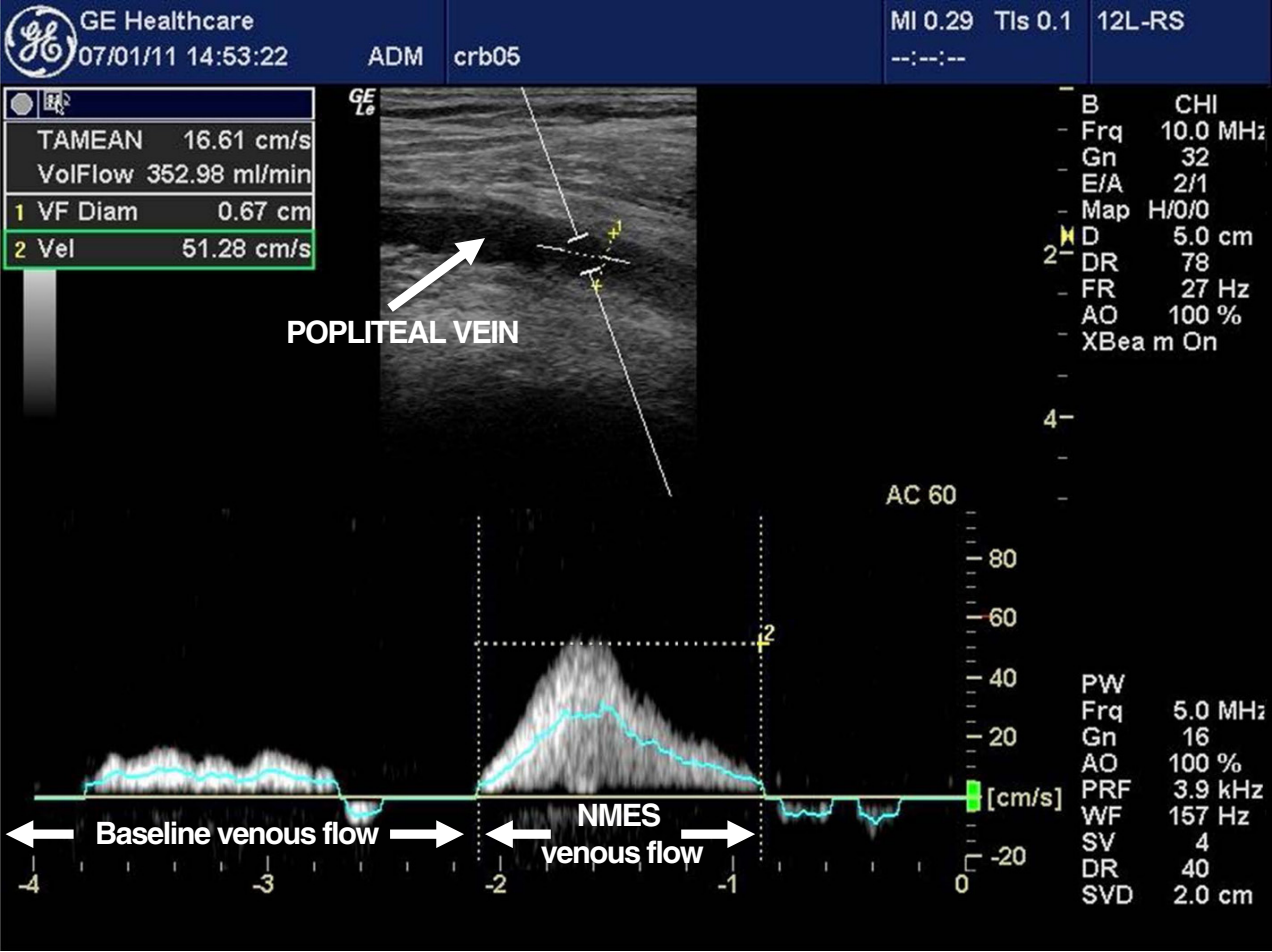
A screenshot of the Doppler machine showing baseline blood flow followed by NMES elicited blood flow.
